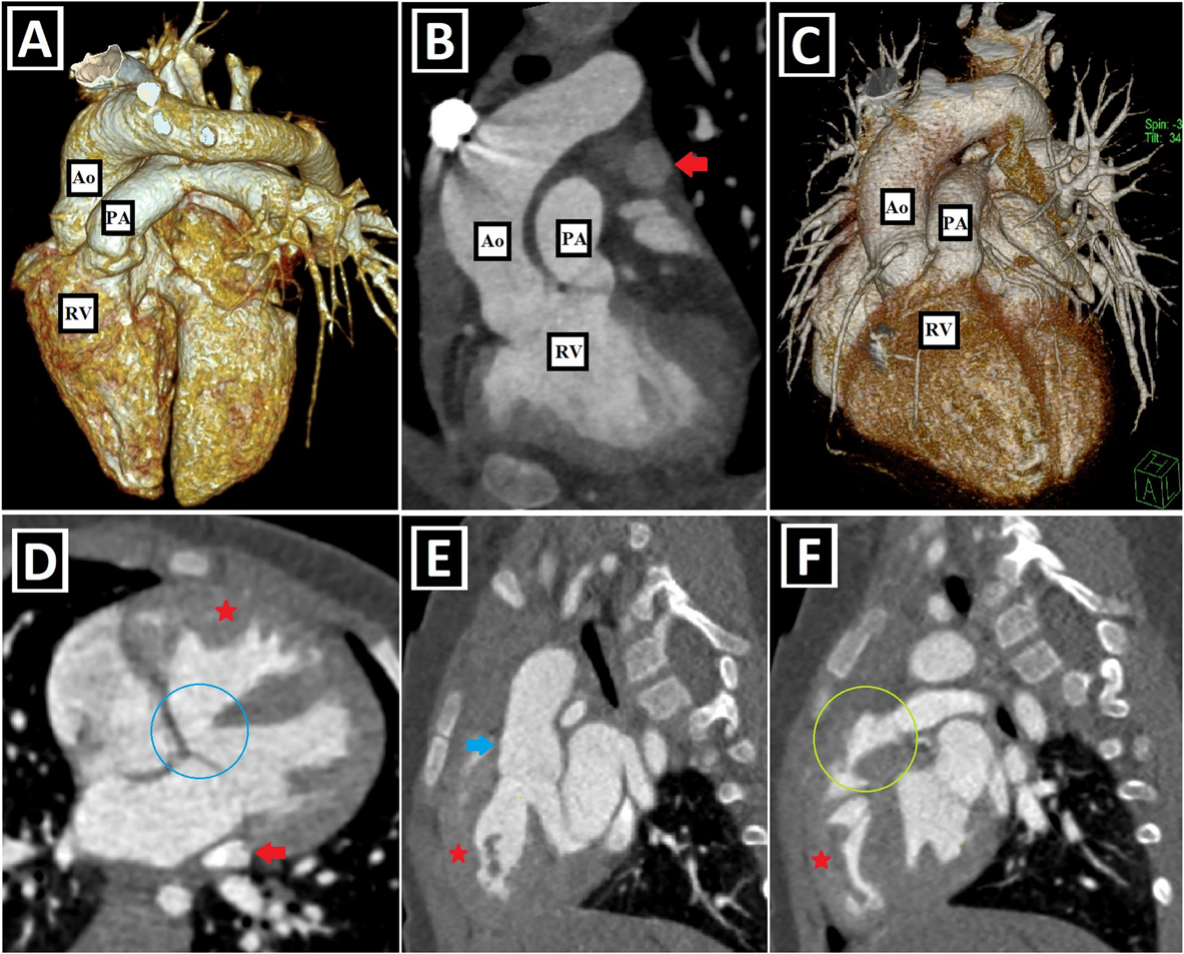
Conotruncal malformations accompanying PLSVC-1. a–c 3D VRT (a, c) and coronal-oblique reformatted (b) CT images show DORV characterized by great arteries (pulmonary artery: PA and aorta: Ao) arising primarily from the right ventricle (RV) in a patient with PLSVC (red arrow). d–f Axial (d) and sagittal-oblique reformatted (e, f) CT images depict TOF characterized by the combination of the right ventricular hypertrophy (red stars), subaortic VSD (blue circle), overriding aorta (blue arrow), and pulmonary stenosis (green circle) in a patient with PLSVC (red arrow)
